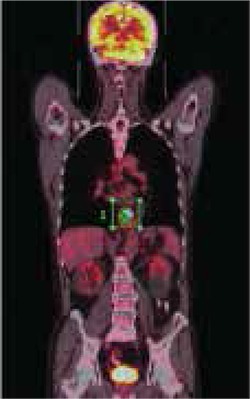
67 year old male patient who had been diagnosed as squa- mous cell cancer by endoscopic biopsy of distal esophageal lesion. 18F-FDG PET/CT showed FDG uptake in distal esophageal lesion with 17.9 SUV_max_ and MTV was calculated as 22.22 cm^3^.
